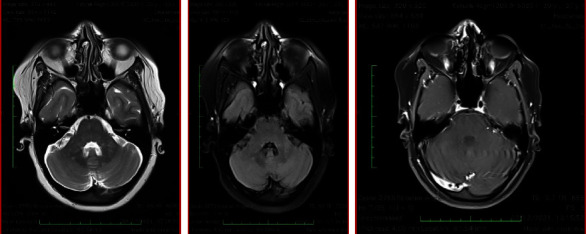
MRI brain images of the same patient, post-treatment (methylprednisolone, azathioprine, and hydroxychloroquine), showing resolution of the lesions in comparison to the previous MRI films.
